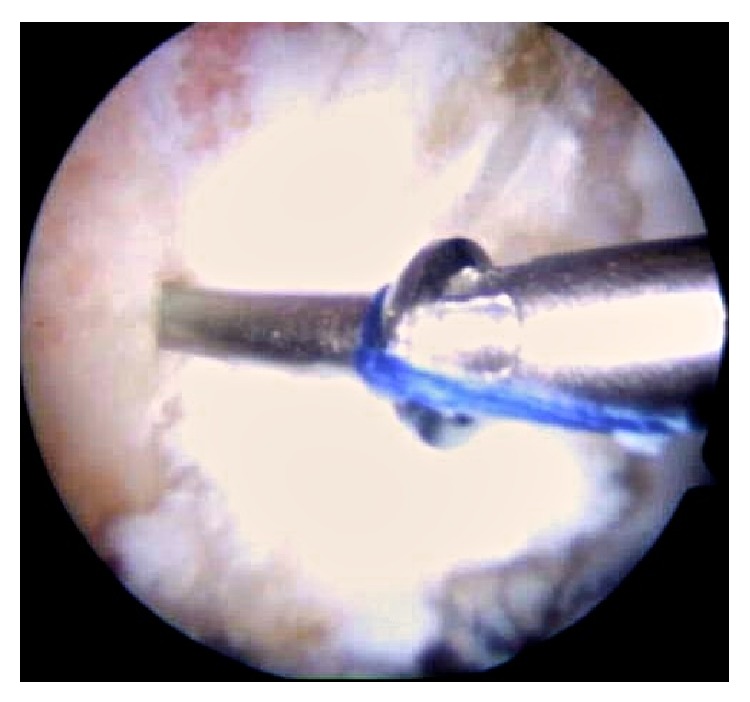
Under X-ray supervision, the remaining bone fragment was secured with an arthroscopic assisted 3.0 mm cannulated screw in both sides.
